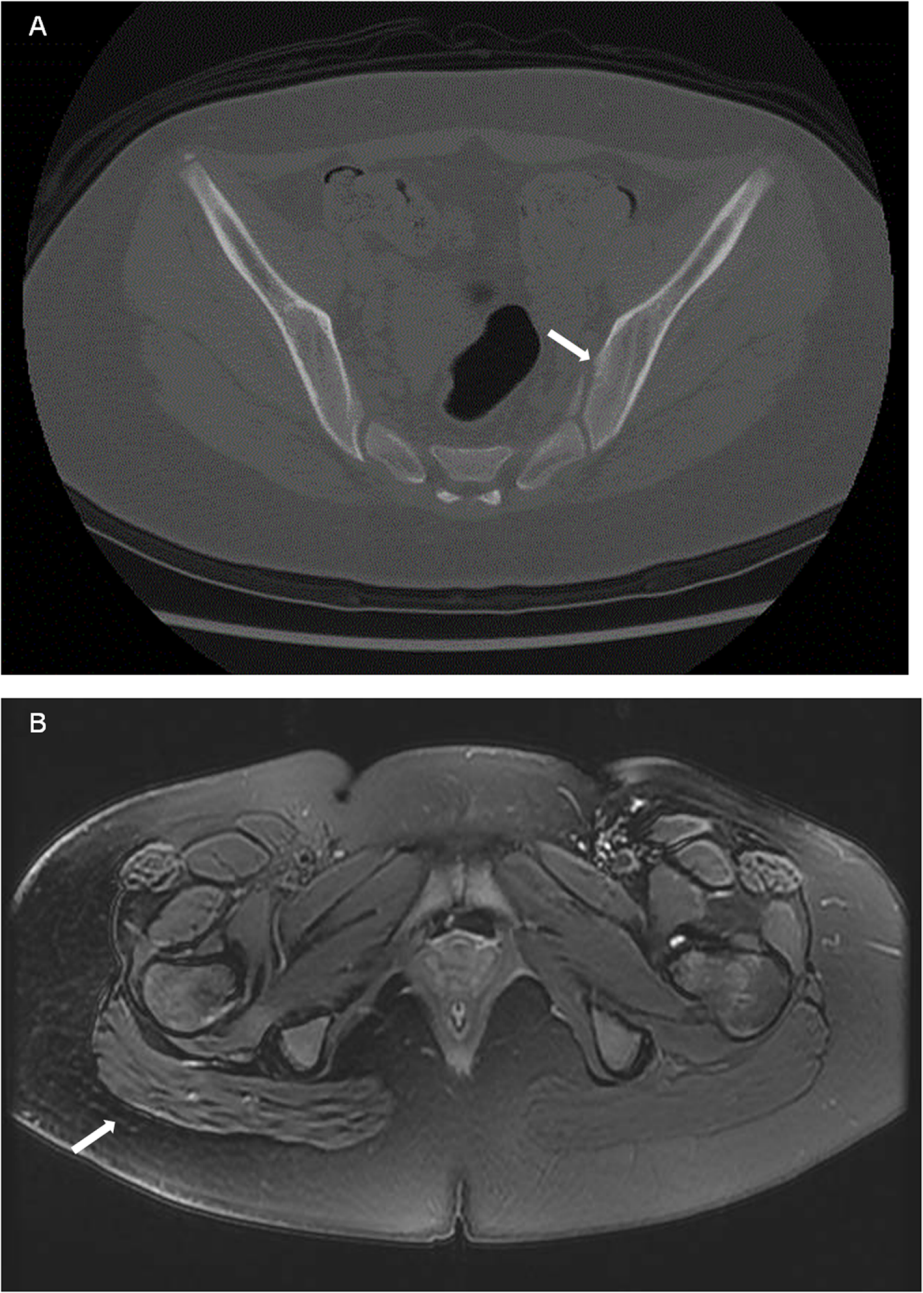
Imagines after treatment. CT revealed no further progression of bone destruction in the SIJ (A), and MRI of hip showed inflammation of the fat layer on the buttocks was significantly reduced (B)
